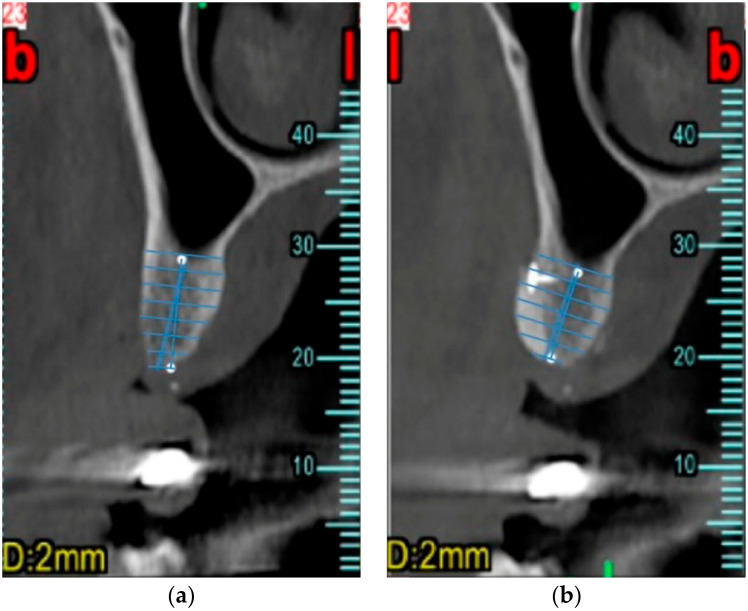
Example of bone thickness measurement. A CBCT section of the crest was selected for each implant site. A line 13 mm in length was drawn along the direction of the ideal implant axis. Perpendicular lines to the implant axis were then identified, marking the variation in bone crest thickness every 1.5 mm. The length of each of these lines was measured. (a) Measurement at baseline (T0); (b) Measurement after 6 months (T1).
